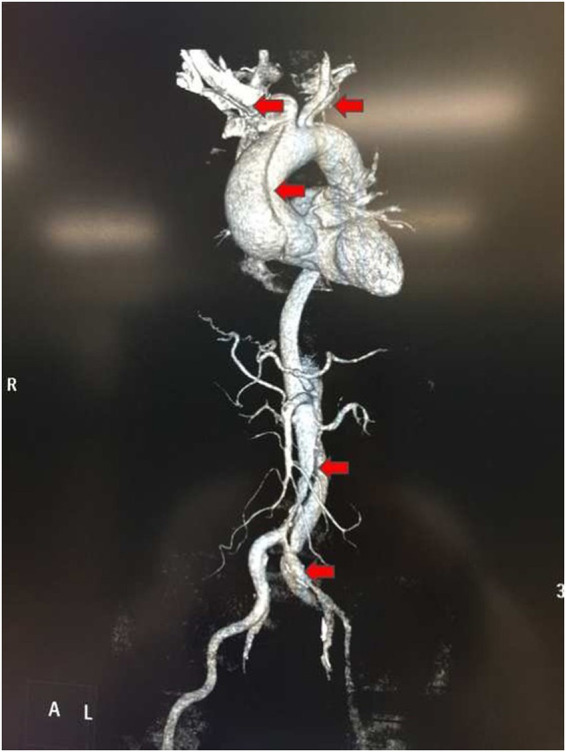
Reconstructed image of computed tomography angiography (CTA). Arrows indicate the dissection of the aortic wall and the false lumen of the DeBakey type I aortic dissection extending from the aortic root to the left external iliac artery.
